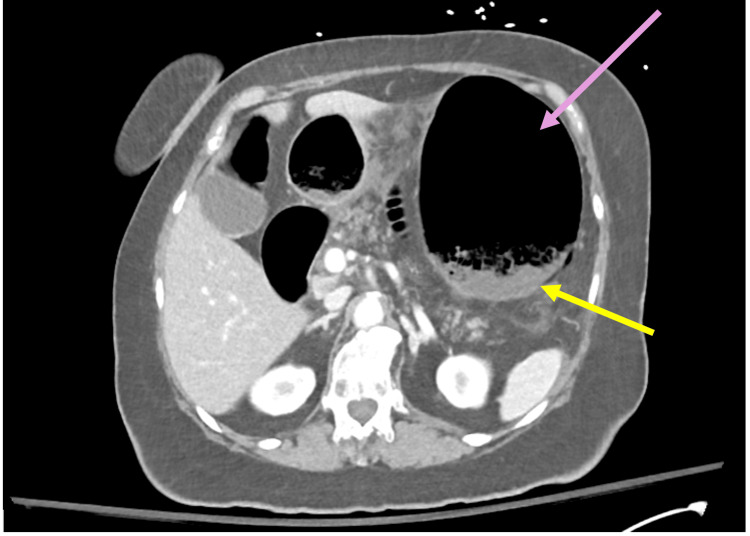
Computed tomography demonstrating gastric pneumatosis and stomach dilation. Purple arrow points to gastric dilation. Yellow arrow points to pneumatosis.
